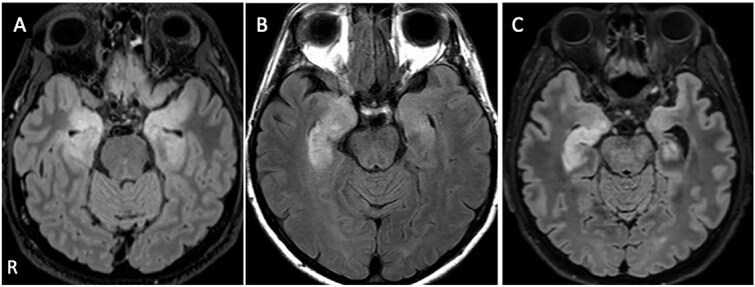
Axial FLAIR images at onset for the three patients revealing hyperintensities in the hippocampus and limbic system. (A) On the left, the axial FLAIR image of Patient 1 shows abnormal hyperintensity of both amygdala-hippocampal complexes, extending to the left basifrontal cortex and left insula. (B) In the middle, the axial FLAIR image of Patient 2 shows asymmetrical hyperintensity in both amygdala-hippocampal complexes, predominant on the right side, with atrophy of the left hippocampus. (C) On the fight, the axial FLAIR image of Patient 3 shows bilateral hyperintensity of amygdala-hippocampal complexes, predominant on the right side. R: right. Other images as well as follow-ups are presented in Supplementary Material 1.
